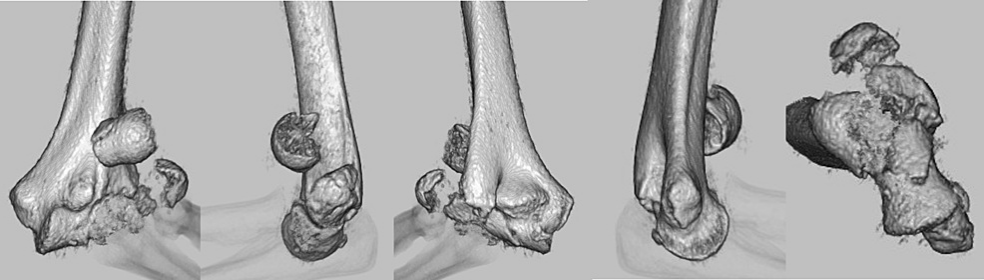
A preoperative three-dimensional computed tomography. Anterior view, radial view, posterior view, ulnar view, and articular surface.
